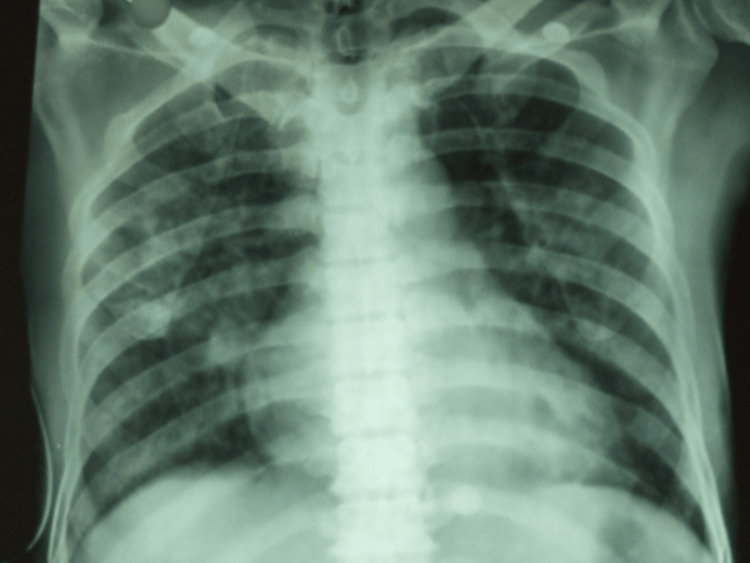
Chest X-ray with bilateral ground glass opacities
